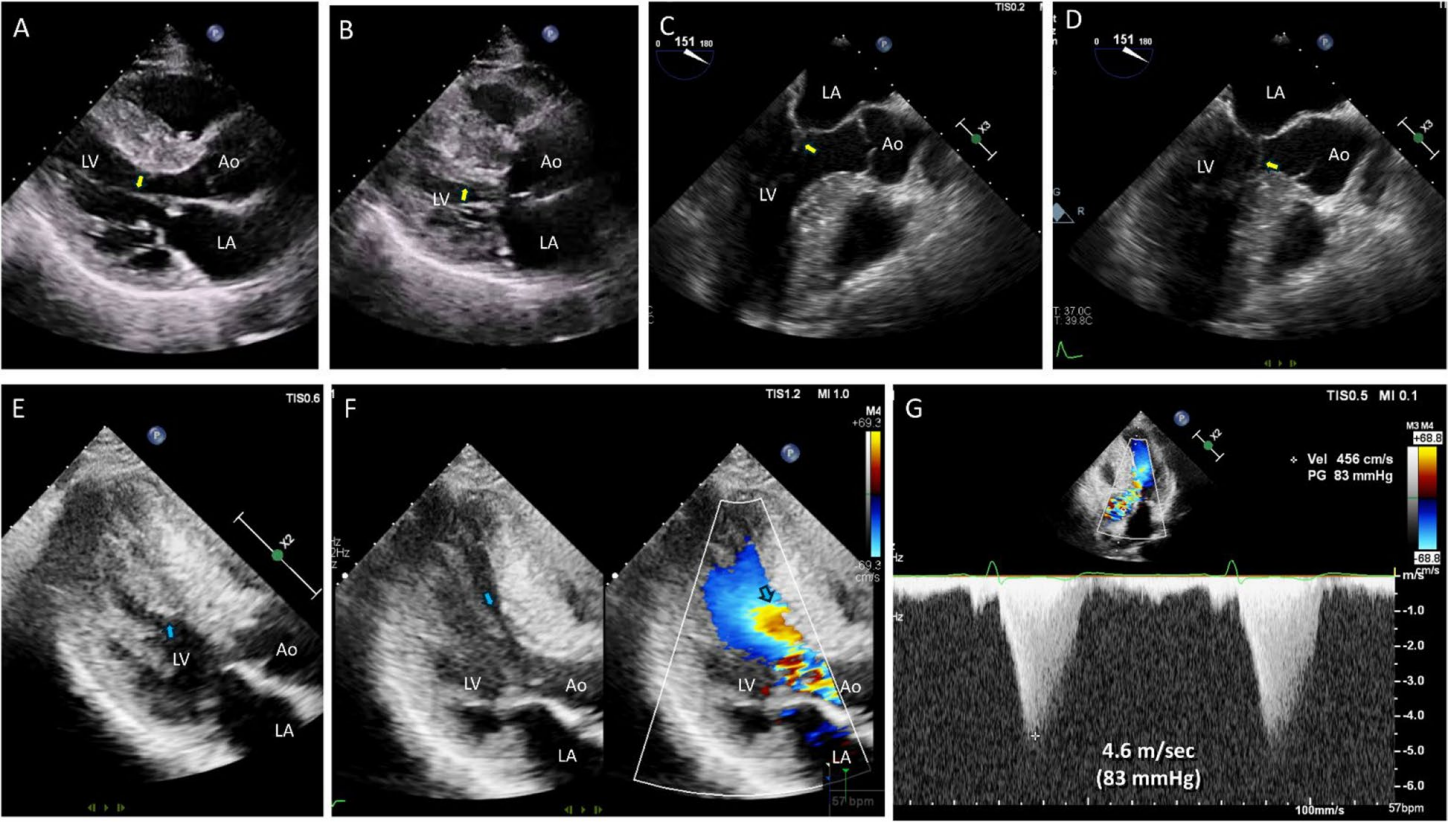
Representative echocardiographic images showing both systolic anterior motion of the mitral valve and elongated papillary muscle causing significant outflow tract obstruction. A, B In the parasternal long-axis view, a thick and elongated papillary muscle with a thin chordae tendineae insertion that contacts a hypertrophied septum during systole is observed (yellow arrows). C, D Transesophageal echocardiography shows systolic anterior motion of the mitral valve with septal contact (yellow arrows). E, F Tilting apical views show anterior movement of the thick and elongated papillary muscle during systole, resulting in flow acceleration (blue arrows). G Doppler tracing shows typical dagger-shaped systolic velocity with a delayed peak
